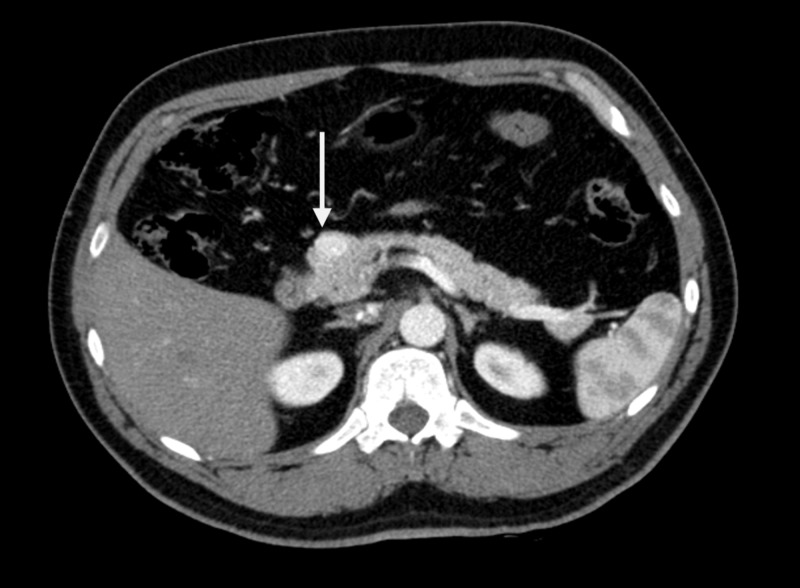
CT Scan of Abdomen Axial computed tomography image demonstrating hyperdense mass lesion at the pancreatic head (arrow).
